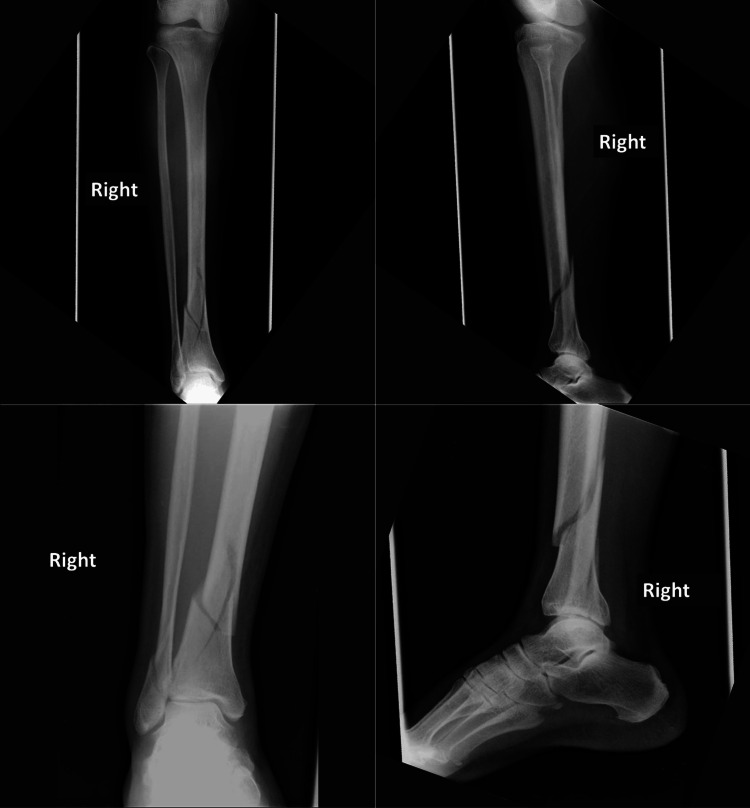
A 43-year-old female patient presented following a road traffic accident with trauma to the right leg and ankle. Anteroposterior and lateral radiographs of the leg revealed a distal quarter tibial fracture with intra-articular extension associated with a fracture of the lateral malleolus. Image credit: Authors
